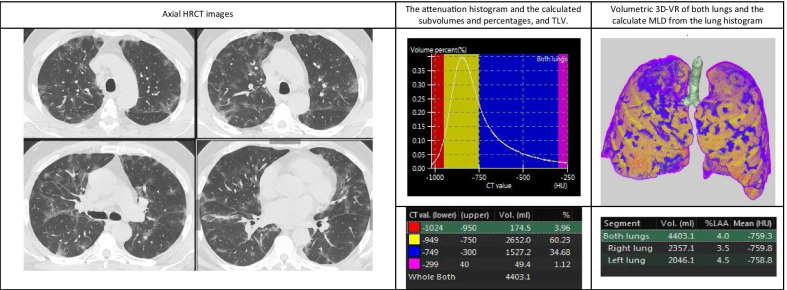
Quantitative CT analysis of COVID-19 pneumonia in 53-year-old man with moderate symptoms that required intermediate ICU admission with high-flow oxygenation support. Axial HRCT images show multiple bilateral relatively large sub-pleural GGOs affecting all lobes. The blue and pink areas in volumetric 3D-VR reflect GGOs and consolidation. The patient had 35.8% disease burden
